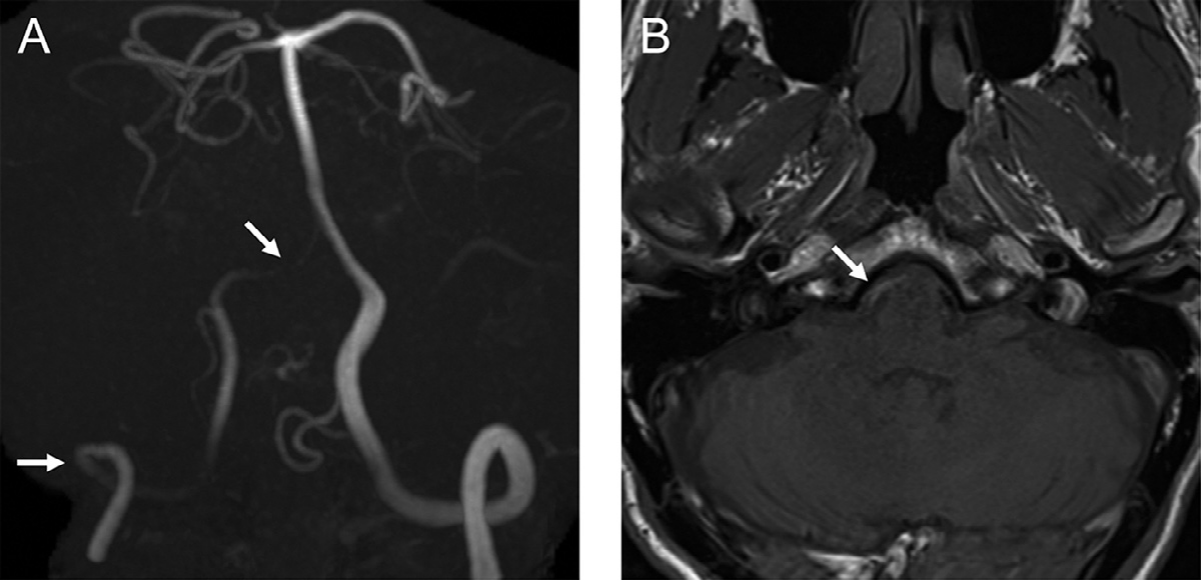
Intracranial vertebral artery dissection diagnosed by magnetic resonance angiography and magnetic resonance imaging. Magnetic resonance angiography showing the poor delineation of the entire right vertebral artery (arrows) (A). T1-weighted image demonstrating an intramural hematoma in the right intracranial vertebral artery distal to the posterior inferior cerebellar artery (arrow) (B).
